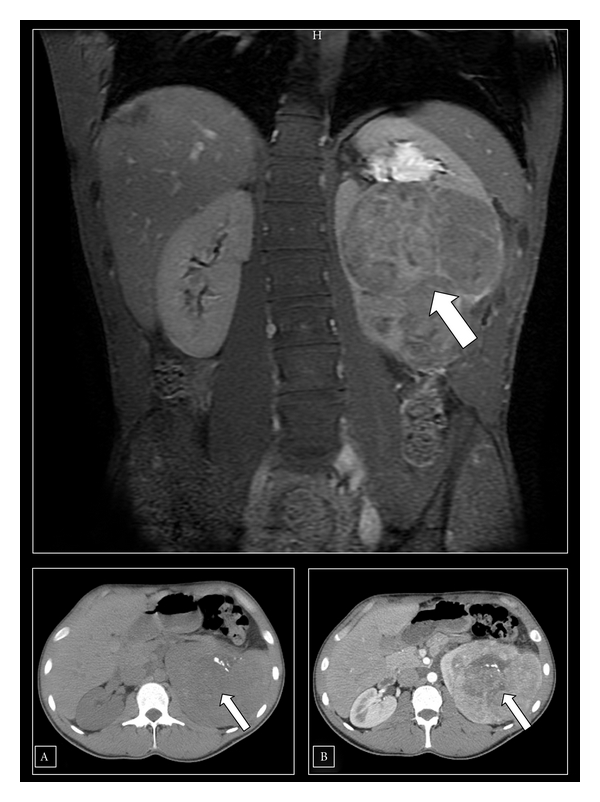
MRI and CT scan (inset (a) without IV contrast and (b) with IV contrast) of the abdomen and pelvis showing a large heterogeneously enhancing (inset (b)) exophytic left renal mass measuring 11.6 × 10.7 cm with multiple small calcifications (arrow inset (a)).
